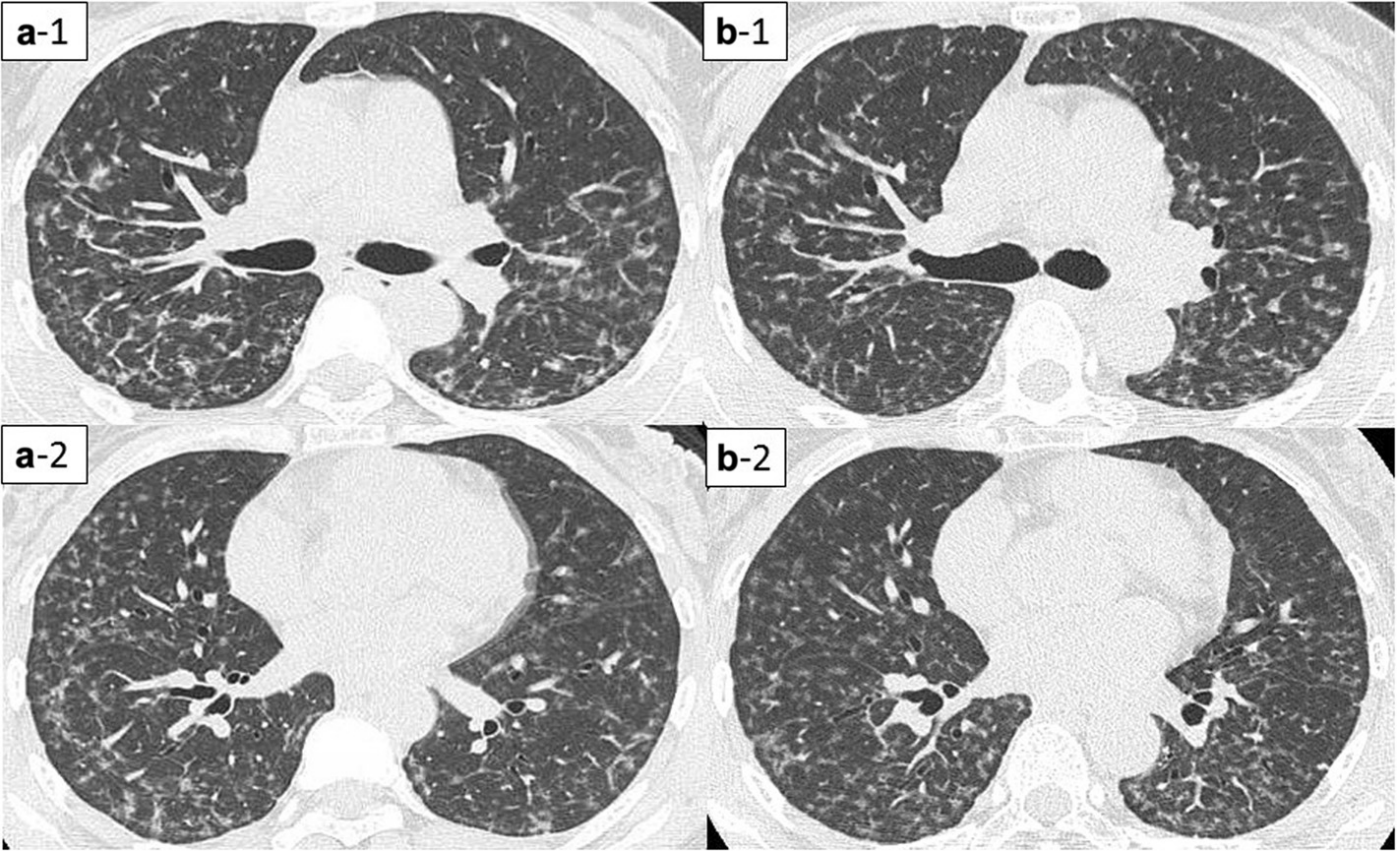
High-resolution computed tomography (HRCT) findings before and after the avoidance of dust exposure in Case1. Bilateral diffuse centrilobular micronodular opacities and curved linear shadows in the dorsum. a: before the avoidance (a-1: upper lobe; a-2: lower lobe); b: after the avoidance (b-1: upper lobe; b-2: lower lobe)
